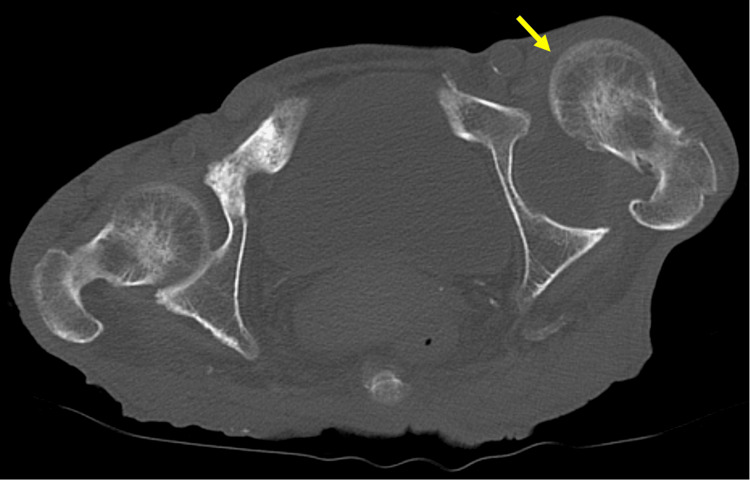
First computed tomography (CT) scan. The first CT scan incidentally revealed anterior dislocation of the left hip joint.
